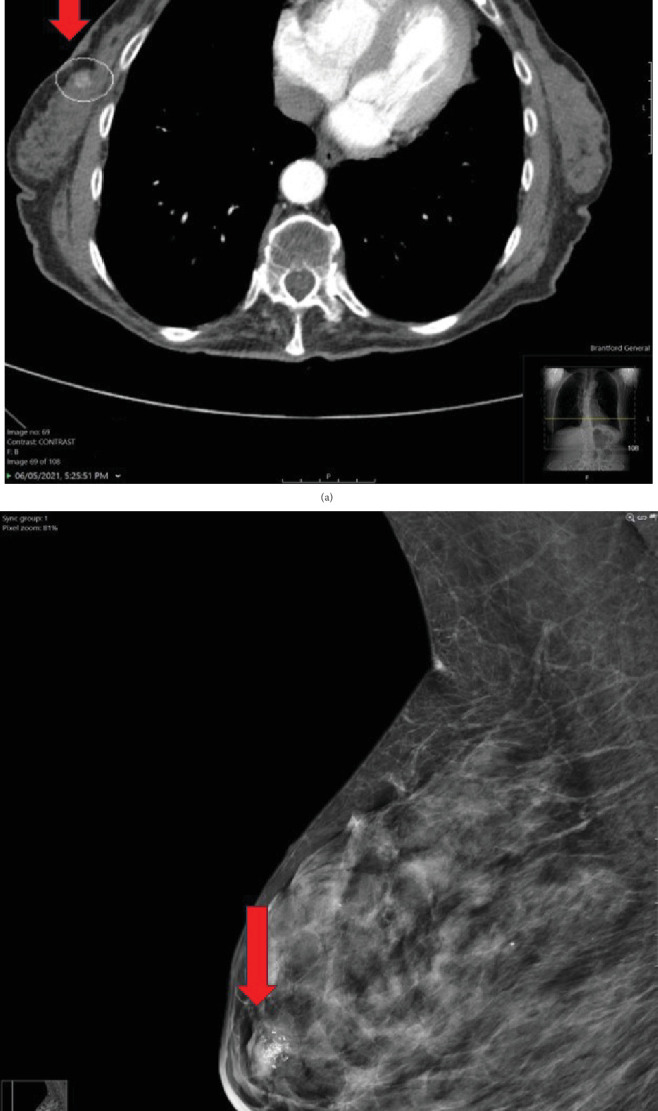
(a) Contrast-enhanced CT of the thorax performed to evaluate pulmonary nodule incidentally demonstrated a 1 cm nodular focus of increased attenuation within the right breast. (b) Subsequent mammogram demonstrated a partially calcified nodule in the right retroareolar region.
